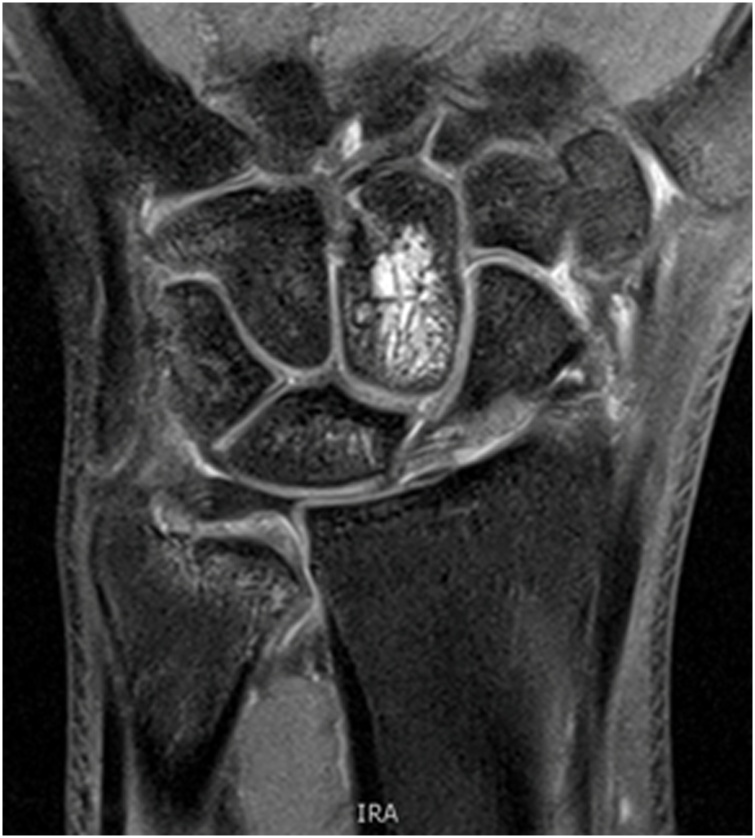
MRI of the patient`s wrist (coronal view).
